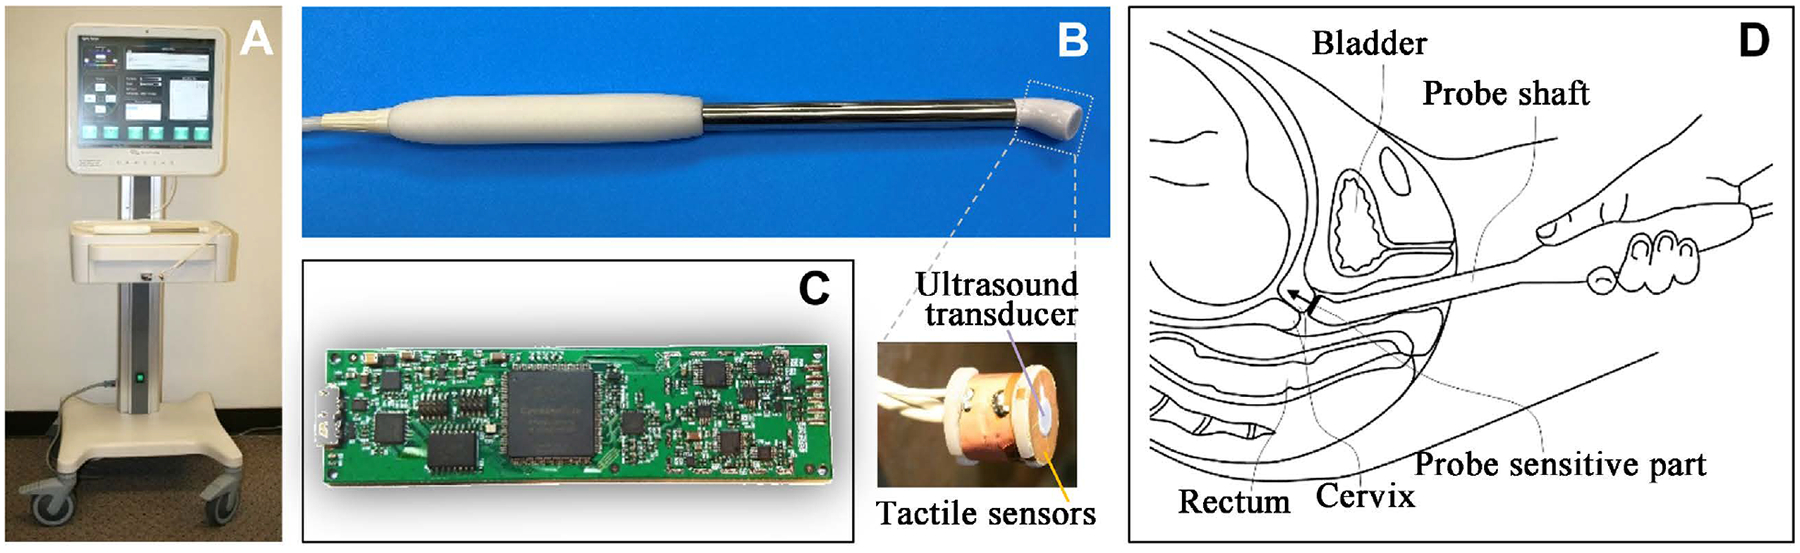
Cervix Monitor design and application. (A) perspective view of the device, (B) cervical probe featuring a single ultrasound transducer and four tactile sensors, (C) printed circuit board designed for data acquisition from both ultrasound and tactile sensors, and (D) cervical probe positioning during data acquisition; the arrow indicates the direction of cervical deformation during measurement.
